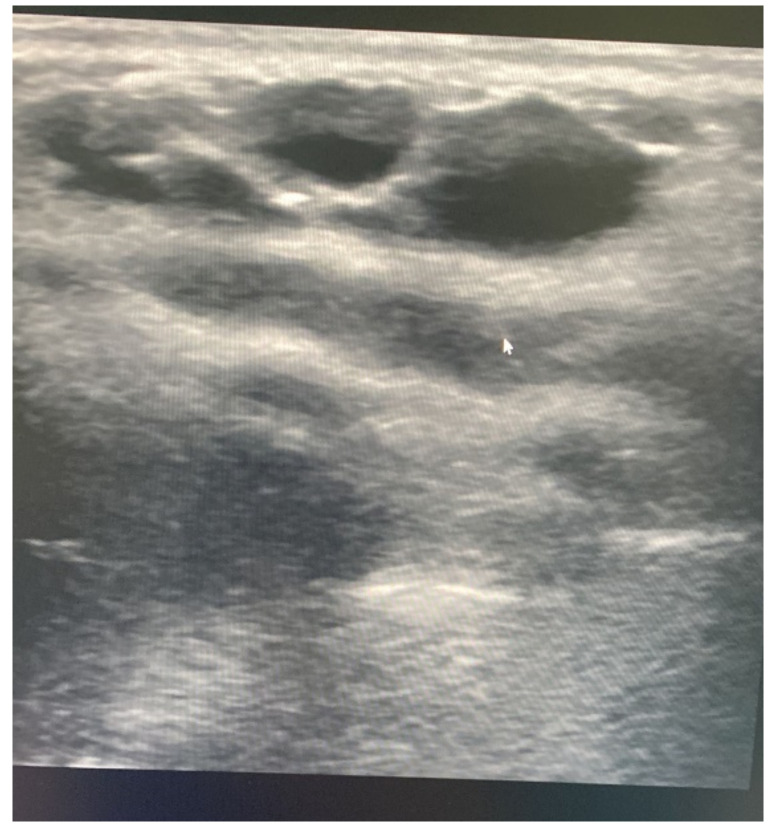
Transverse grey-scale ultrasound image shows an anechoic multilocular cystic lesion in the left lateral chest wall.
